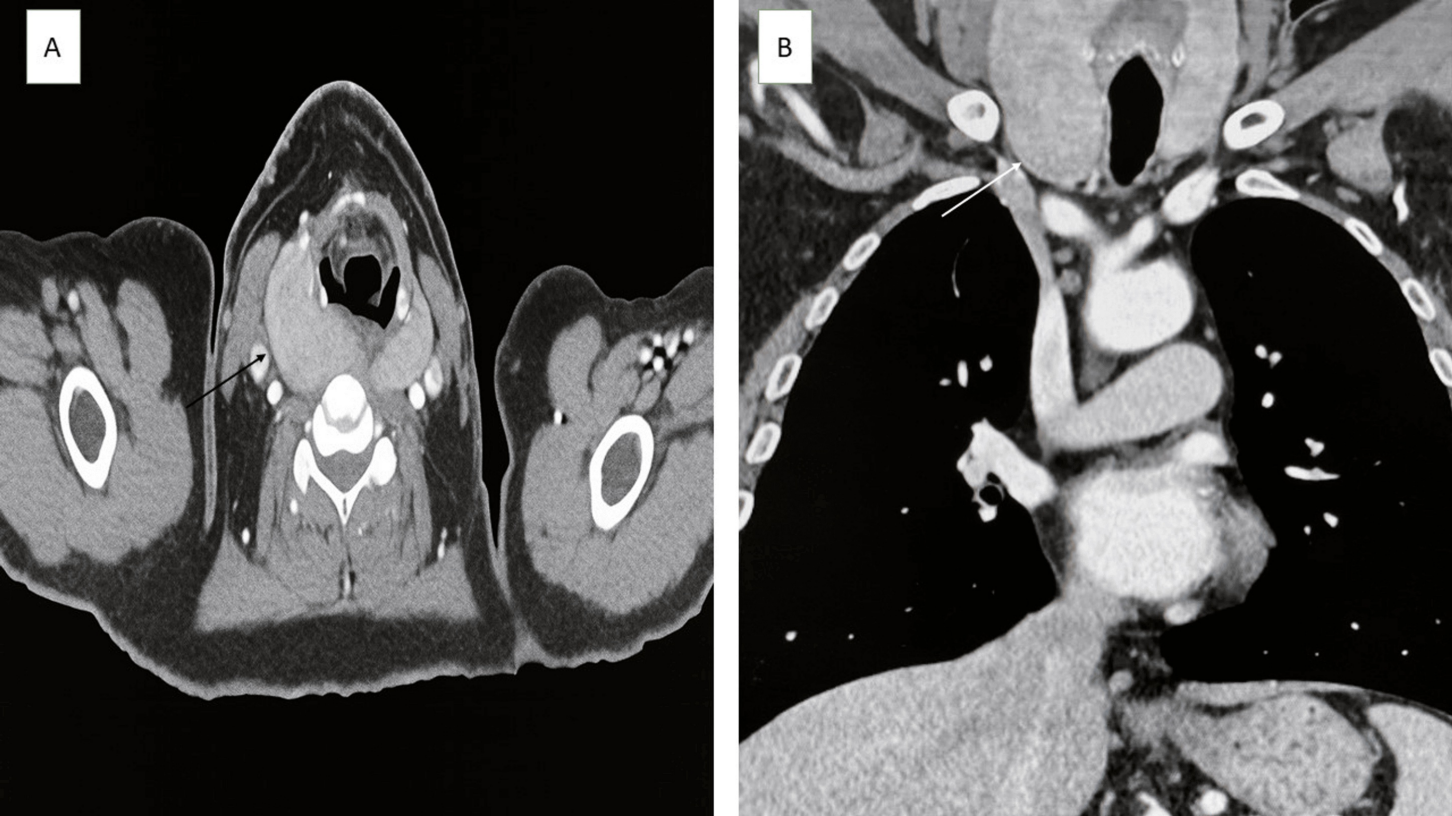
A. Axial CT showing diffusely enlarged thyroid gland (black arrow). B. Coronal CT showing enormous thyroid gland extending to the upper mediastinum (white arrow).
